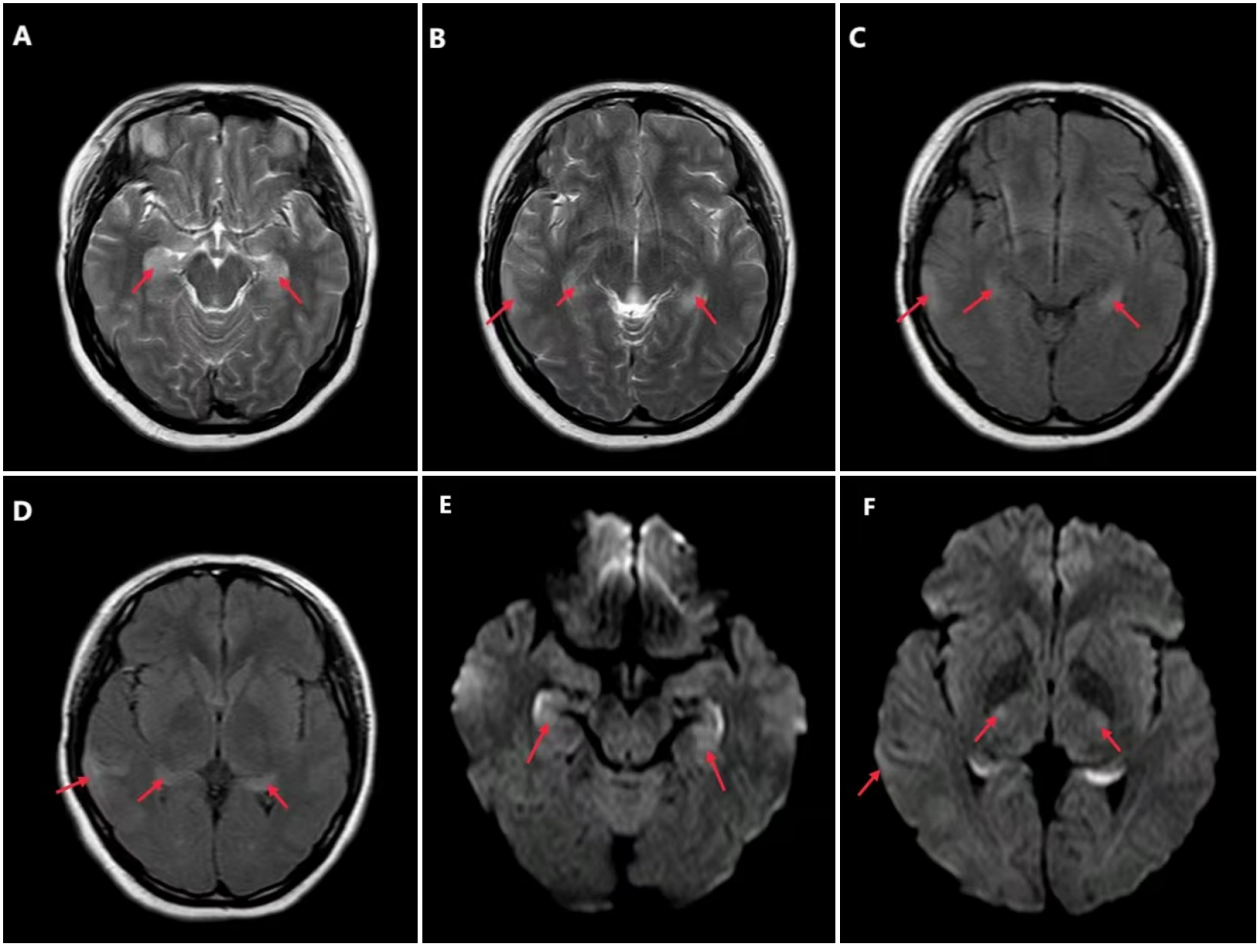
Brain MRI revealed patchy T2WI/FLAIR hyperintensity and abnormally elevated DWI signals in bilateral hippocampus and right temporal parietal lobe (as shown by the arrow). (A, B) T2WI, (T2-weighted imaging); (C, D) FLAIR, (fluid attenuated inversion recovery); (E, F) DWI, (diffusion- weighted imaging).
